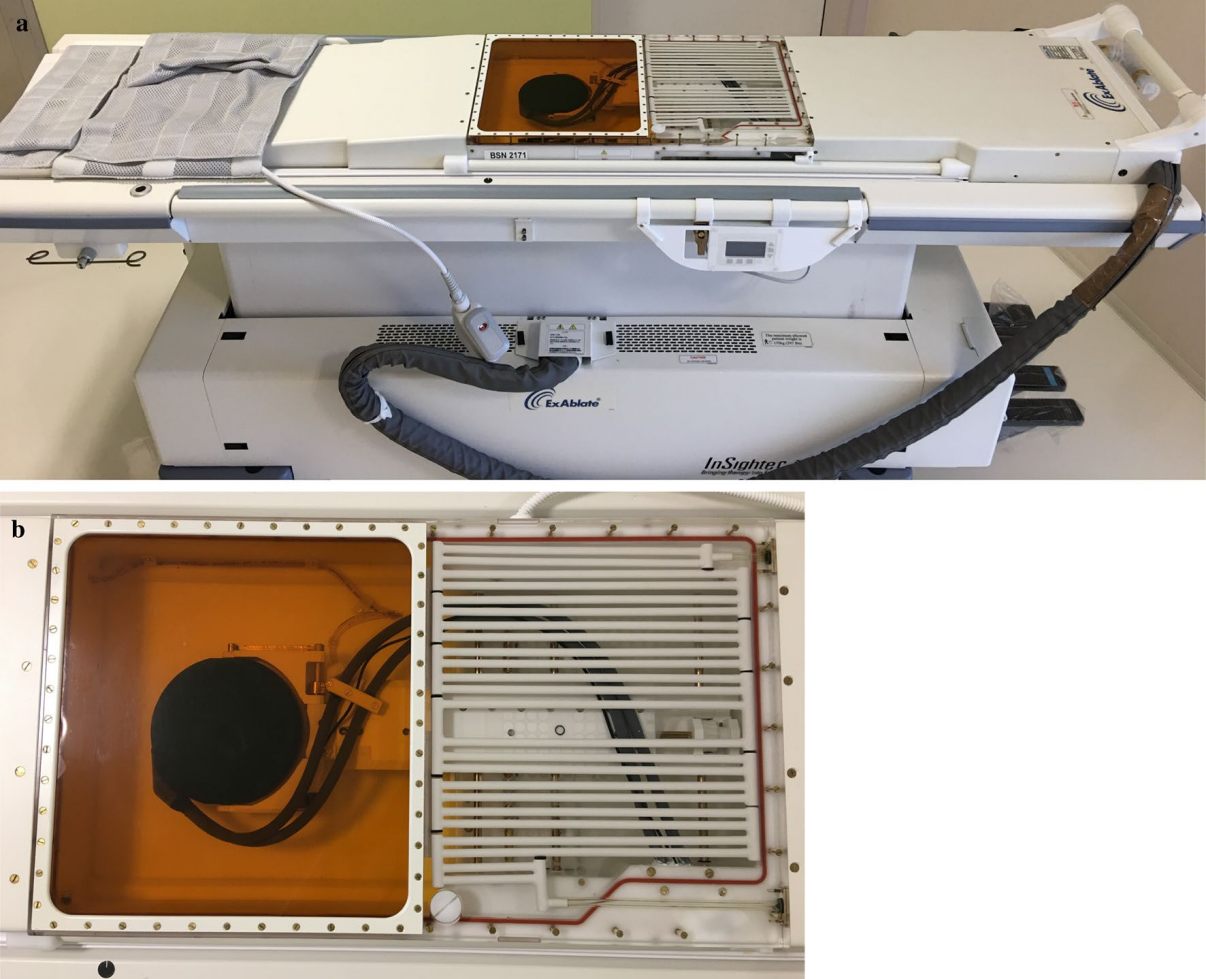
The ExAblate 2100 treatment table. a Photograph shows the patient treatment table. Before treatment, this table is connected to the MRI scanner. b Photograph shows FUS transducer and the positioning device of the FUS transducer. During treatment, the FUS transducer moves to the appropriate area for sonicating the targeted lesion
